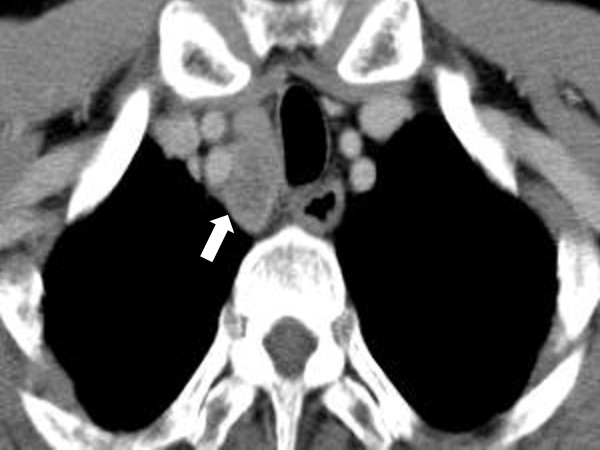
A pre-treatment CT-scan showing metastatic lymph node invasion to the right subclavian artery (arrow).
